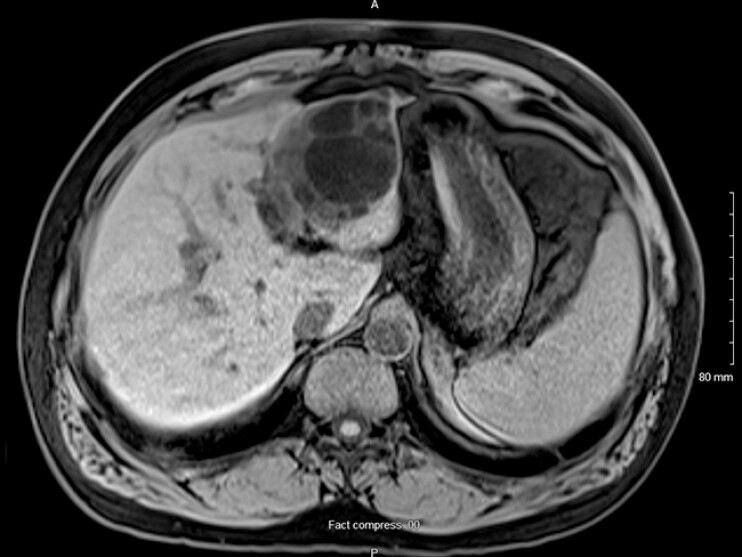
MRI revealed interval growth of the hepatic cyst and left intra-hepatic biliary ductal dilatation. MRI, magnetic resonance imaging.
